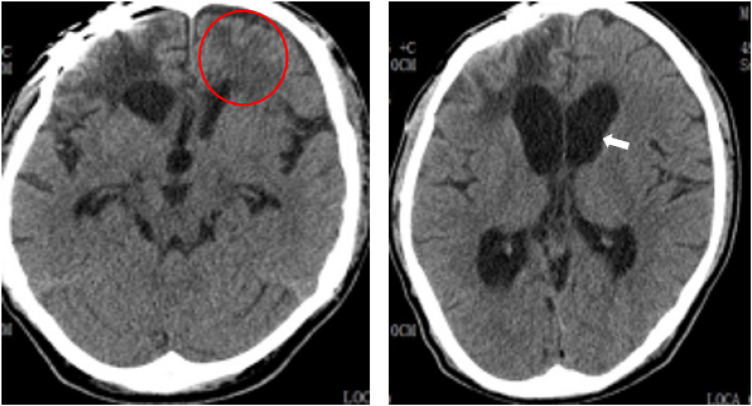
Cranial CT: the brain abscess has resolved (red circle); no progressive hydrocephalus changes are noted (thick arrow).
